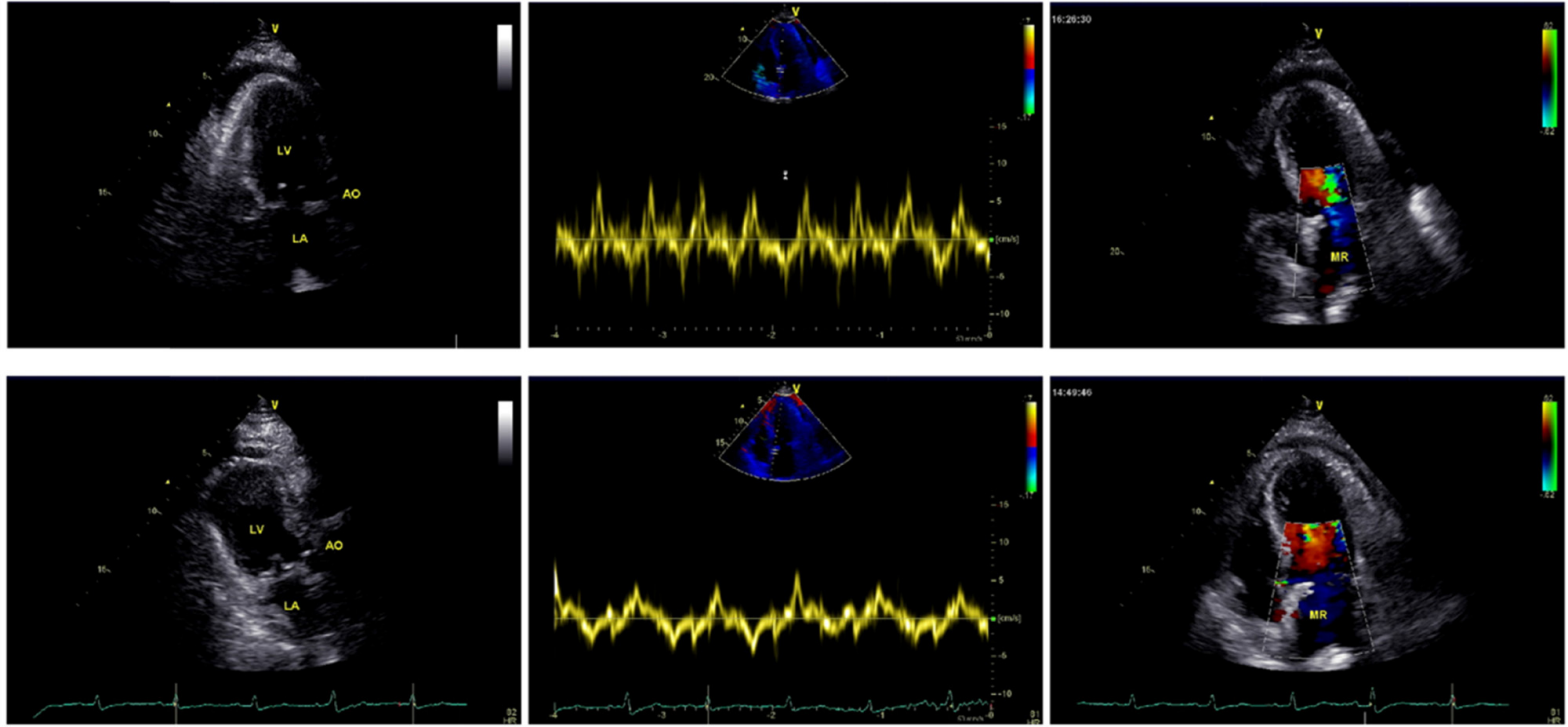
Comparison of cardiac ultrasound findings before and after treatment in a patient (male, 57 years old); the first row is before treatment and the second row is after treatment.
